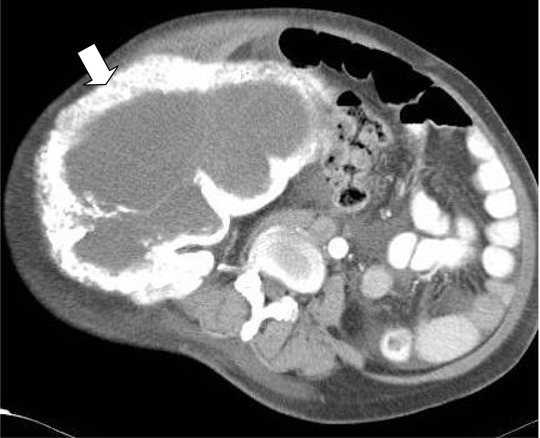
Contrast-enhanced computed tomography of the pelvis and the abdomen showed huge expansile lytic lesion (arrow) arising from the illium and ischium. The bowels are displaced to the left. Small area of soft tissue component (open arrow). The cortex is thickened and irregular. The lower abdomen and the pelvis were tilted by this huge mass.
